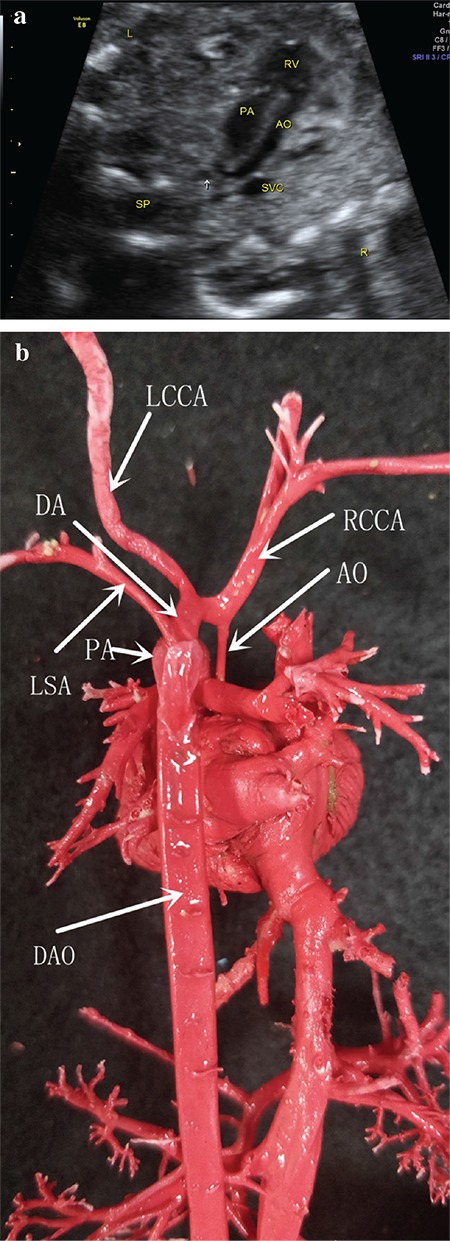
Prenatal ultrasound only diagnosed coarctation of the aortic arch and double outlet of right ventricle (a). The cast shows interruption of the aortic arch and ductus arteriosus abnormal connections (LCCA-DA-PA) (b). AO: Ascending aorta; DA: ductus arteriosus; DAO: descending aorta; LCCA: Left common carotid artery; LSA: Left subclavian artery; PA: pulmonary artery; RCCA: right common carotid artery
